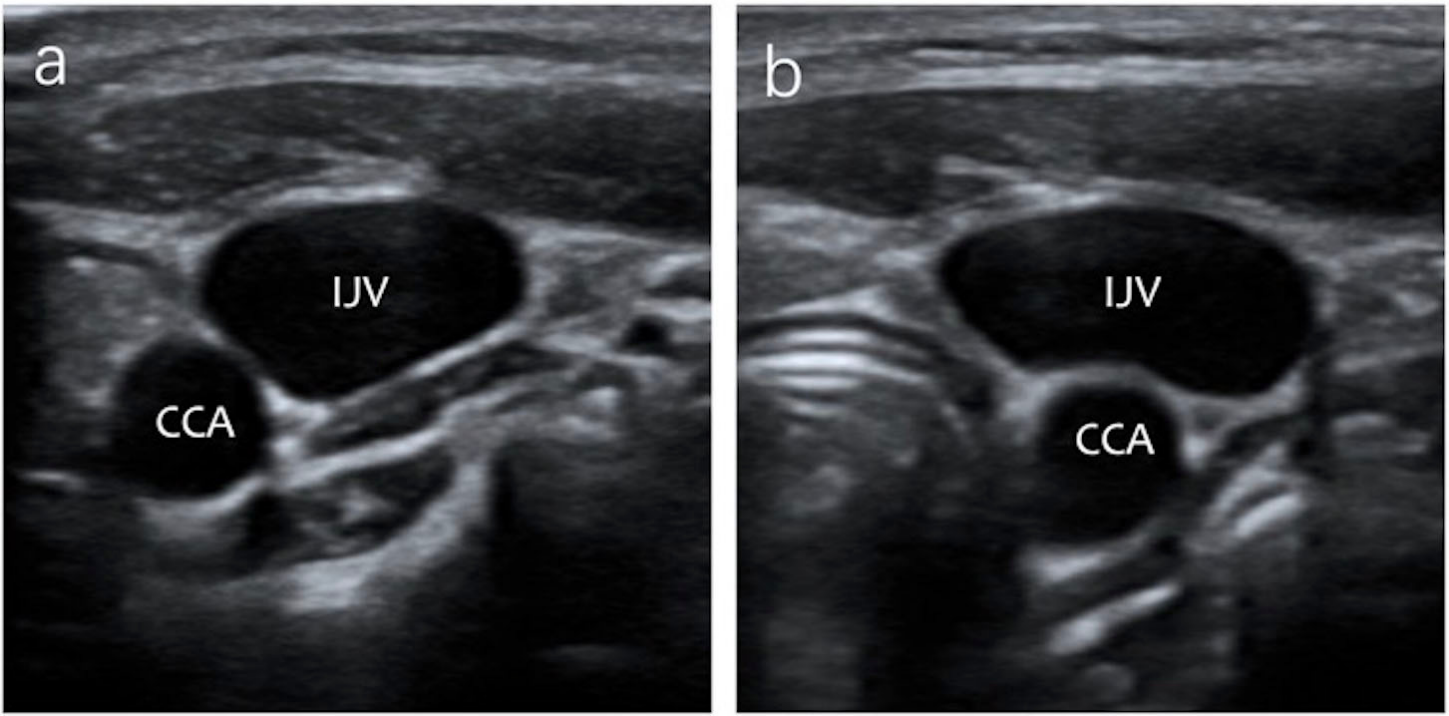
The IJV anatomical position in relationship changed from AL to A in a patient under ultrasound images after LMA insertion. (a) The anatomic position relationship is AL under ultrasonography before LMA insertion. (b) The anatomic position relationship is A under ultrasonography after LMA insertion.
